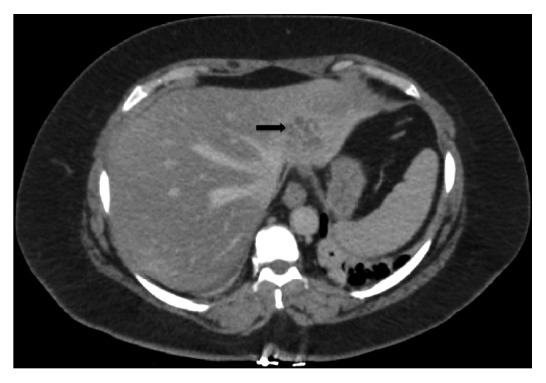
Computed tomography showing 3.2 x 4.0 x 1.8 cm multiseptated cystic lesion in the left hepatic lobe.
